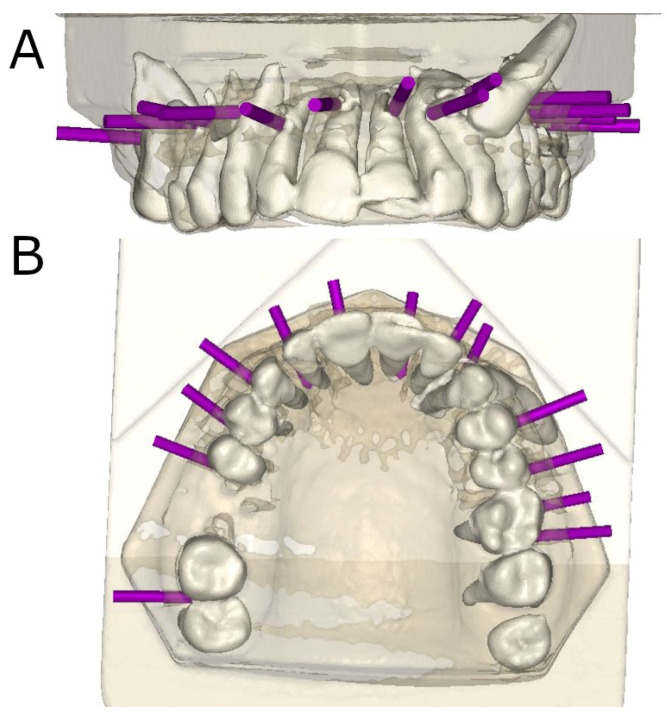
(A) Front view and (B) occlusal view of the postoperatively rendered CBCT scan with the preparations of the osteotomy sites (purple cylinders).
